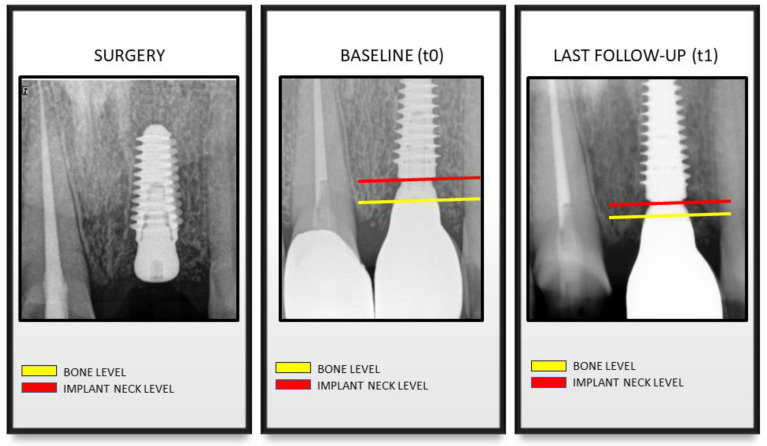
X-rays were taken at surgery, prosthetic delivery (baseline), and at the follow-up visit (t_1_). Measurements of marginal bone level (MBL) were taken for the mesial and the distal aspect of each implant.
